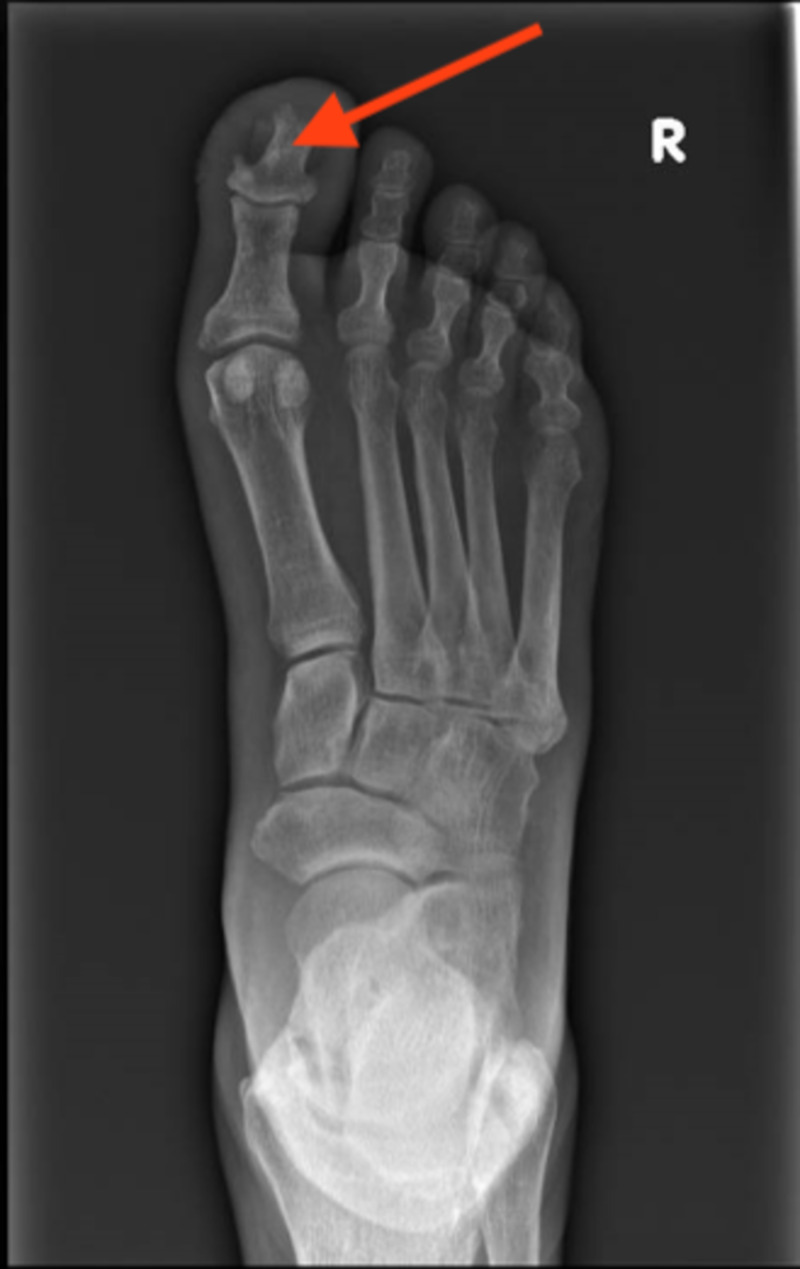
X-ray showing erosion of distal phalanx of the right big toe
